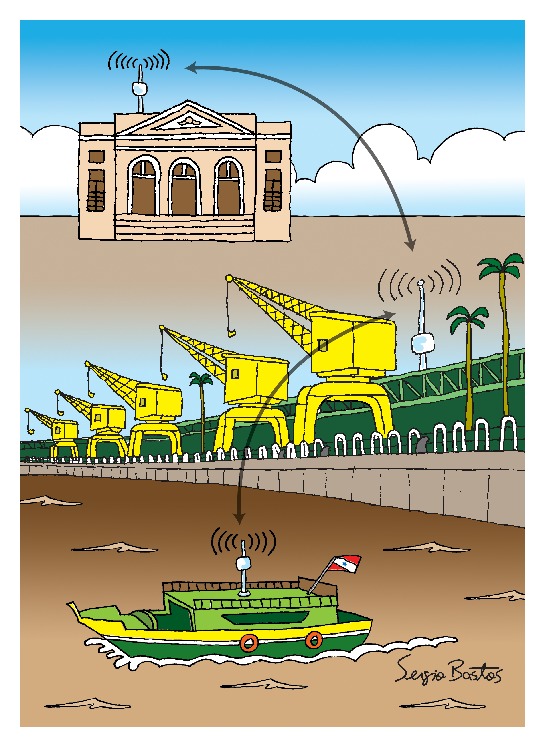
Delivery of ultrasound files at the state capital.
